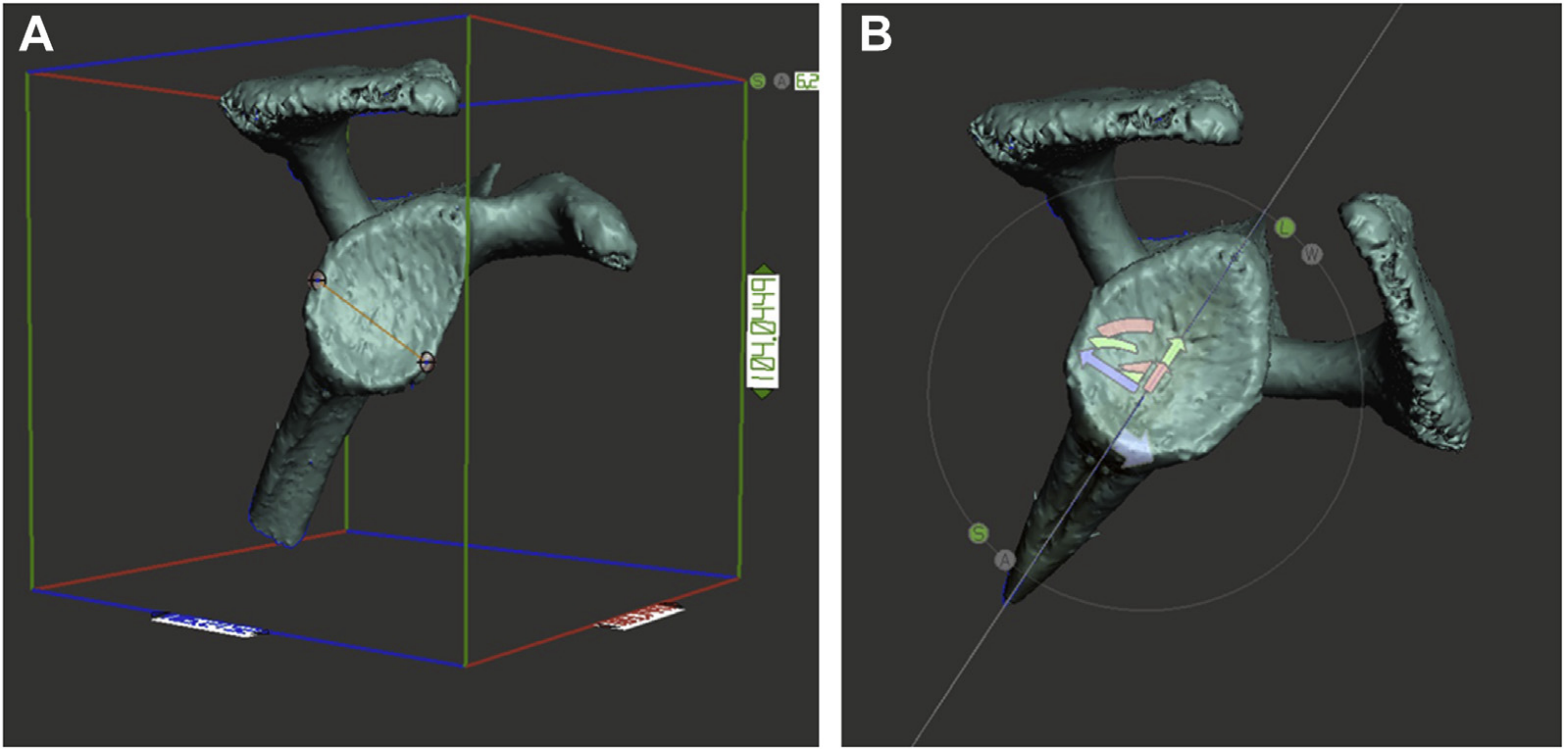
(A) The affected glenoid width is measured on an en face view of a 3-dimensional reconstruction of the CT. (B) The glenoid is mirrored symmetrically in order to measure the unaffected glenoid width.
